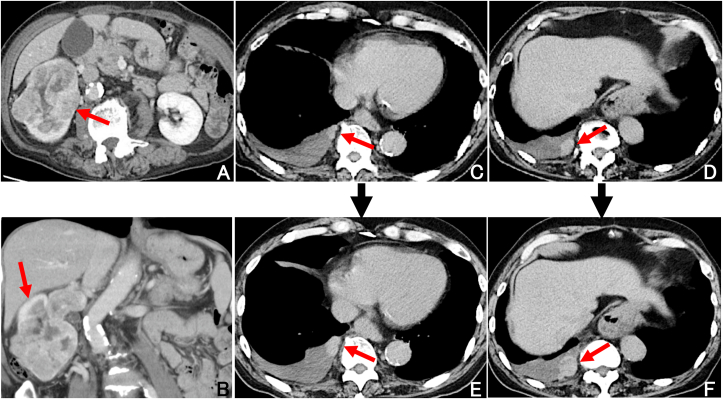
(A, B) Contrast-enhanced CT shows right RCC before nephrectomy. (C, D) Non-enhanced CT shows multiple right pleural tumors with pleural effusion. (E, F) Non-enhanced CT shows rapid growth of the pleural mass after one month.
